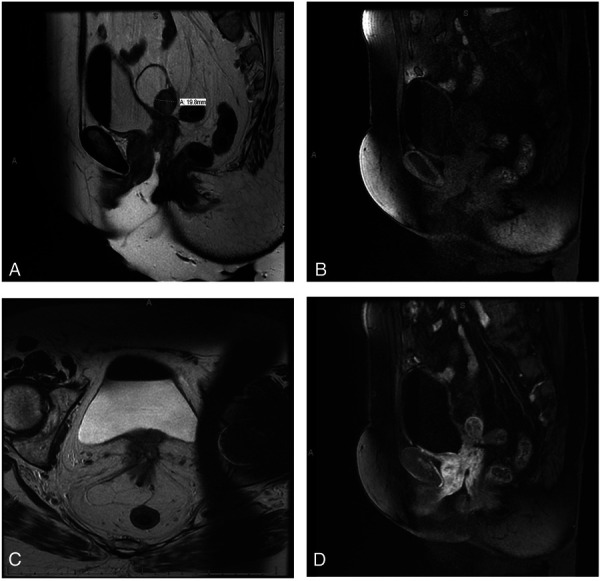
Magnetic resonance imaging of the pelvis showing involvement of ovary and vaginal cuff (A–C) and degree of postcontrast enhancement (D).
